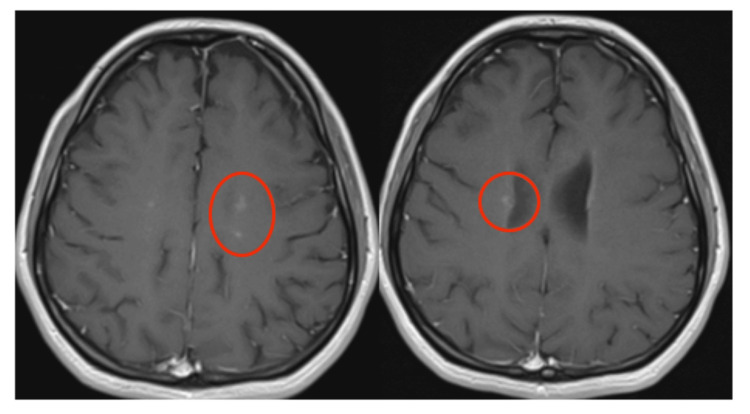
MRI head revealed enhancements (red circles) in post-contrast T1 sequence.
